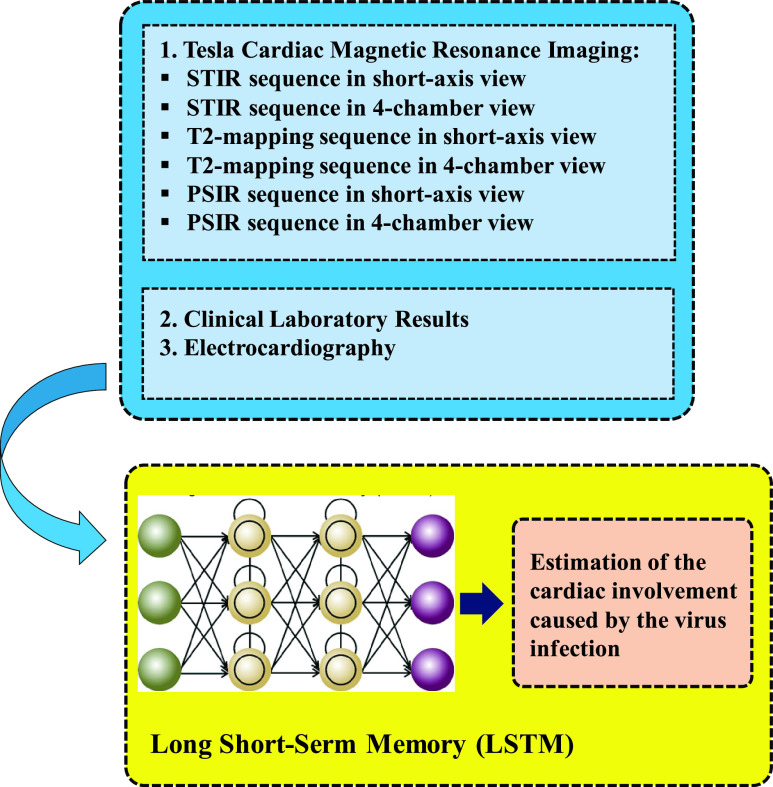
Estimation of cardiac involvement caused by the virus infection extracted from The features from Tesla cardiac magnetic resonance imaging and the information given in [64].
